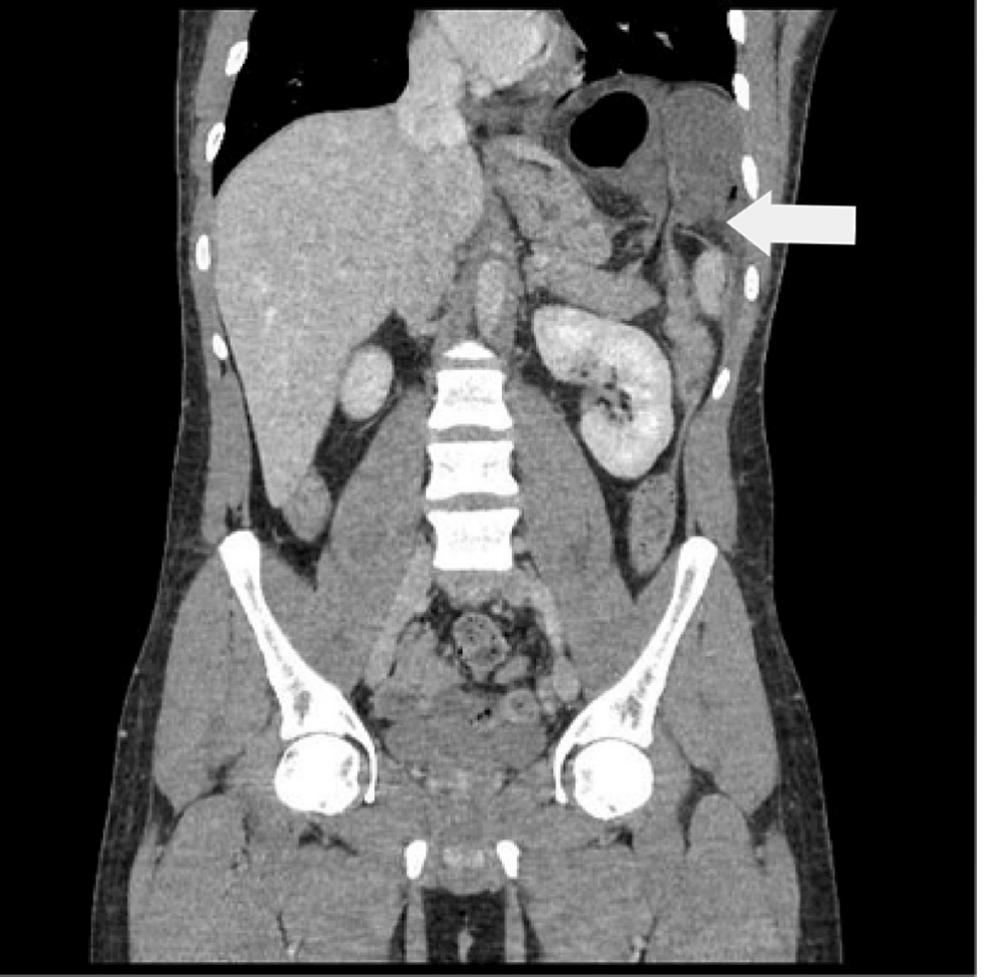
Computed tomography showing a left diaphragmatic hernia containing dilated small bowel loops with mesentery and omentum (white arrow)
